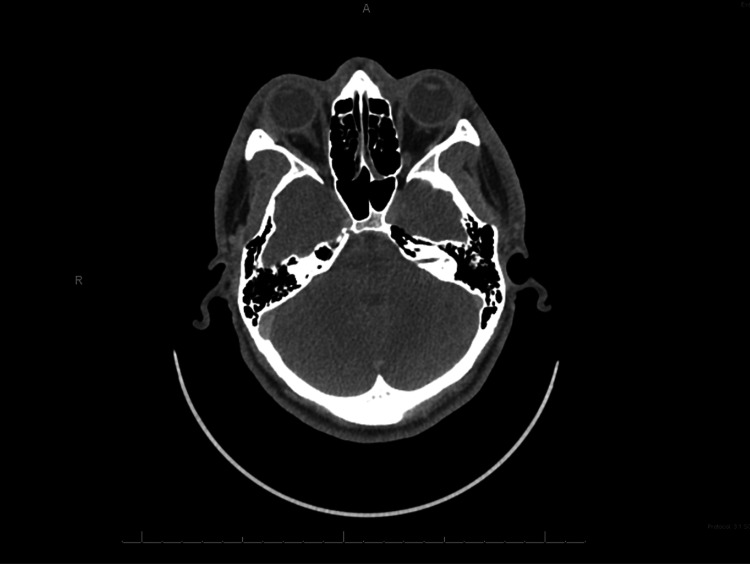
CT of the head and neck showing dermal thickening and subjacent subcutaneous stranding through the anterior face consistent with cellulitis.
